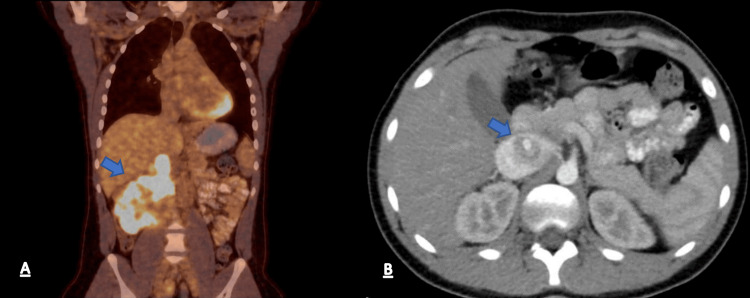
Preoperative PET/CT scan showing (A) increased 18F-FDG uptake involving the right retroperitoneum and inferior vena cava (arrow) and (B) tumor thrombus in inferior vena cava (arrow).
